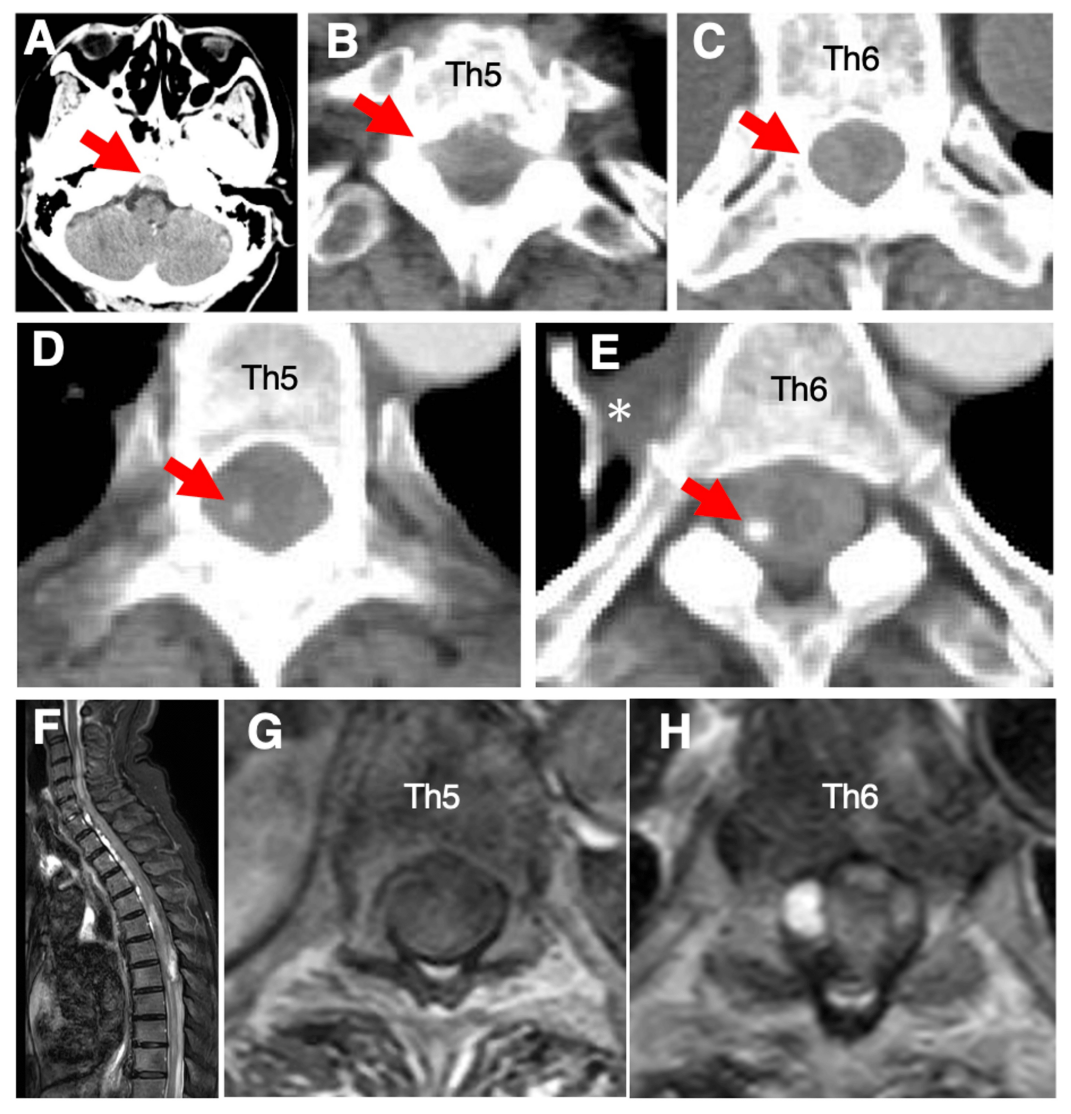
Imaging findings of a case of acute spinal subdural hematoma (A–C) CT images from the previous hospital revealed a hematoma (red arrows) extending from the epidural space of the clivus to the spinal canal of the cervical and thoracic spinal cord (A: head axial; B: Th5 axial; C: Th6 axial). (D, E) Contrast-enhanced CT obtained six hours after symptom onset showed a spot sign at the Th5–6 level (red arrows) and an enlarged right intercostal artery (asterisk) (D: Th5 axial; E: Th6 axial). (F–H) MRI performed before admission demonstrated an intraspinal hematoma and intramedullary signal changes in the cervical and thoracic spinal cord (F: sagittal T2-weighted image; G: Th5 axial; H: Th6 axial). Abbreviations: CT, computed tomography; MRI, magnetic resonance imaging; ASSDH, acute spinal subdural hematoma
